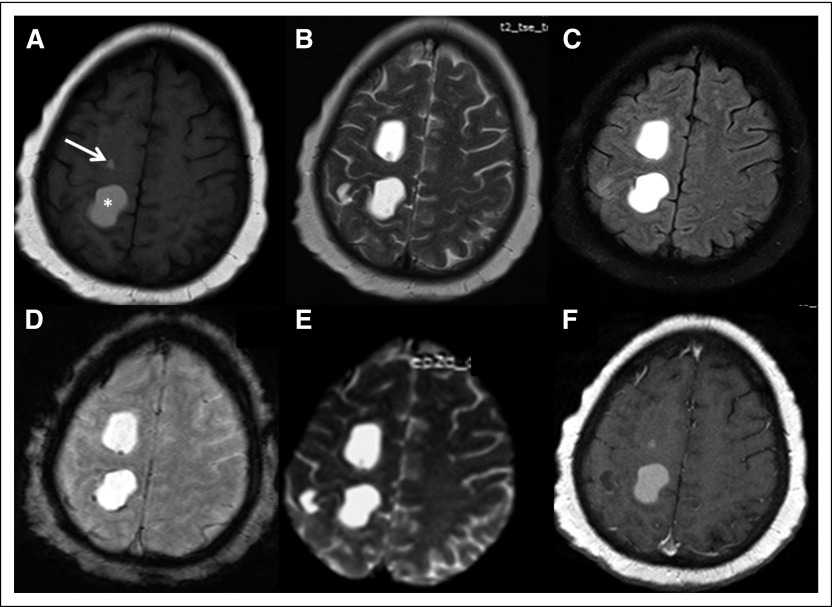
(A) T1-weighted, (B) T2-weighted, (C) fluid-attenuated inversion recovery, (D) susceptibility-weighted, (E) *m*-ADC (mean apparent diffusion coefficient) map, and (F) postcontrast T1-weighted magnetic resonance images showing multiple cystic lesions in the right cerebral hemisphere. The cyst followed fluid signal on magnetic resonance imaging (hypointense to isointense relative to CSF on T1-weighted images, hyperintense on T2-weighted images, and suppressed on fluid-attenuated inversion recovery images). A few of the cystic lesions were completely hyperintense on T1-weighted images (asterisk), and a few showed eccentric hyperintense focus on T1-weighted imaging (arrow) that on susceptibility-weighted images revealed eccentric focal blooming corresponding to the calcification seen on CT imaging; no hemorrhage was seen within. None of the lesions showed diffusion restriction, perilesional edema, or enhancing solid component within.
